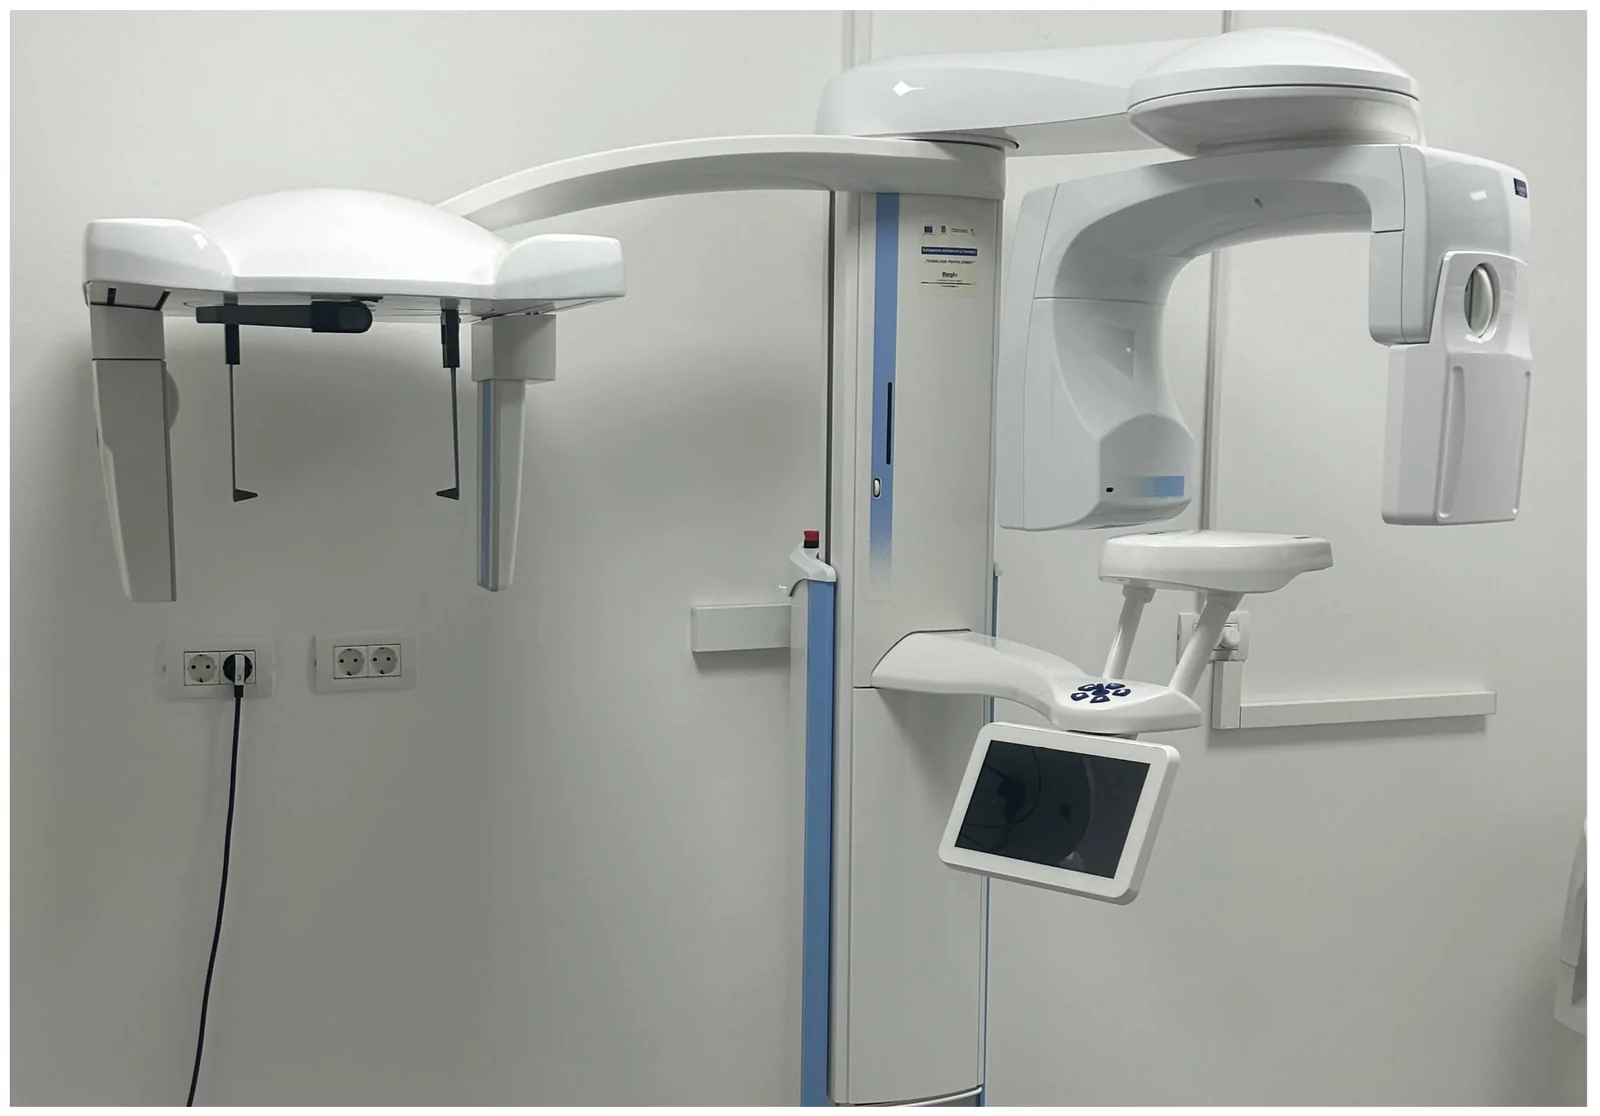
Planmeca ProMax X-ray unit from the dental clinic.
